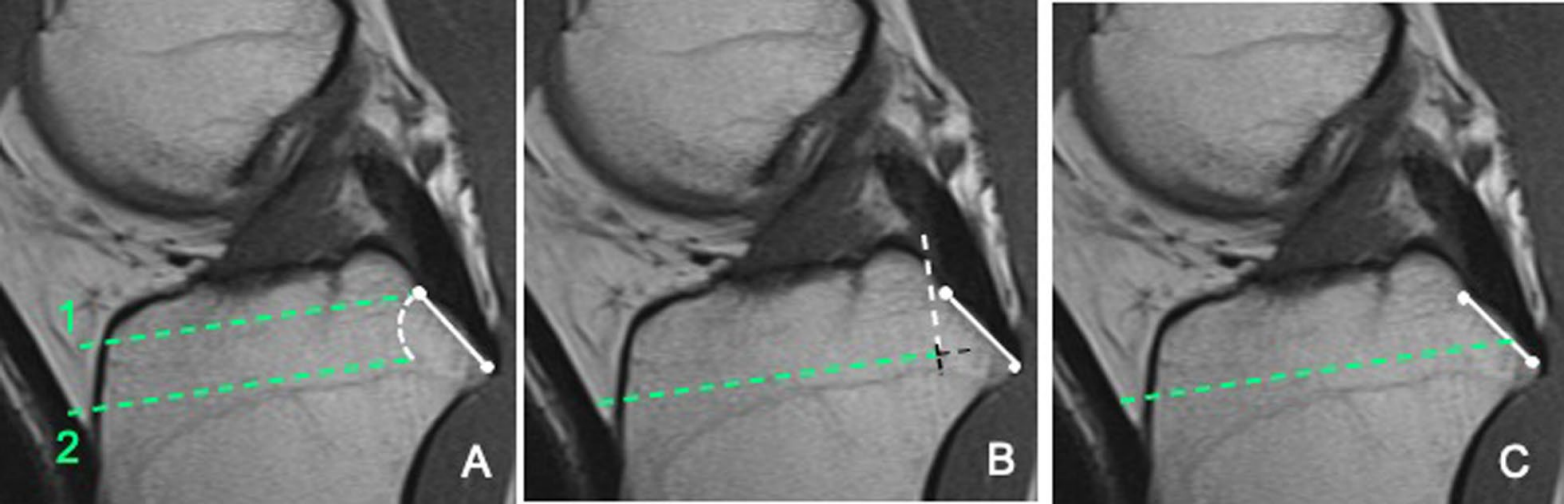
Sagittal MRI scans showing the different techniques of tibial cutting. The white continuous line represents the PCL insertion in the PCL facet. A Double tibial cut technique. The dotted line 1 and 2 represent the first and second tibial cut; the white dotted line represents the residual bone adjacent to the PCL insertion after the second cut. B Bone island technique. The white dotted line represents a limited osteotomy performed to isolate a bone block in front of the PCL. When the bone block is inadvertently undermined by the saw blade (black dotted lines) its anchoring area is reduced leading to increased risks of detachment due to the traction exerted by the PCL. C en bloc resection. The image highlights the risk of damaging, and difficulty in protecting, the PCL insertion when this technique is used
